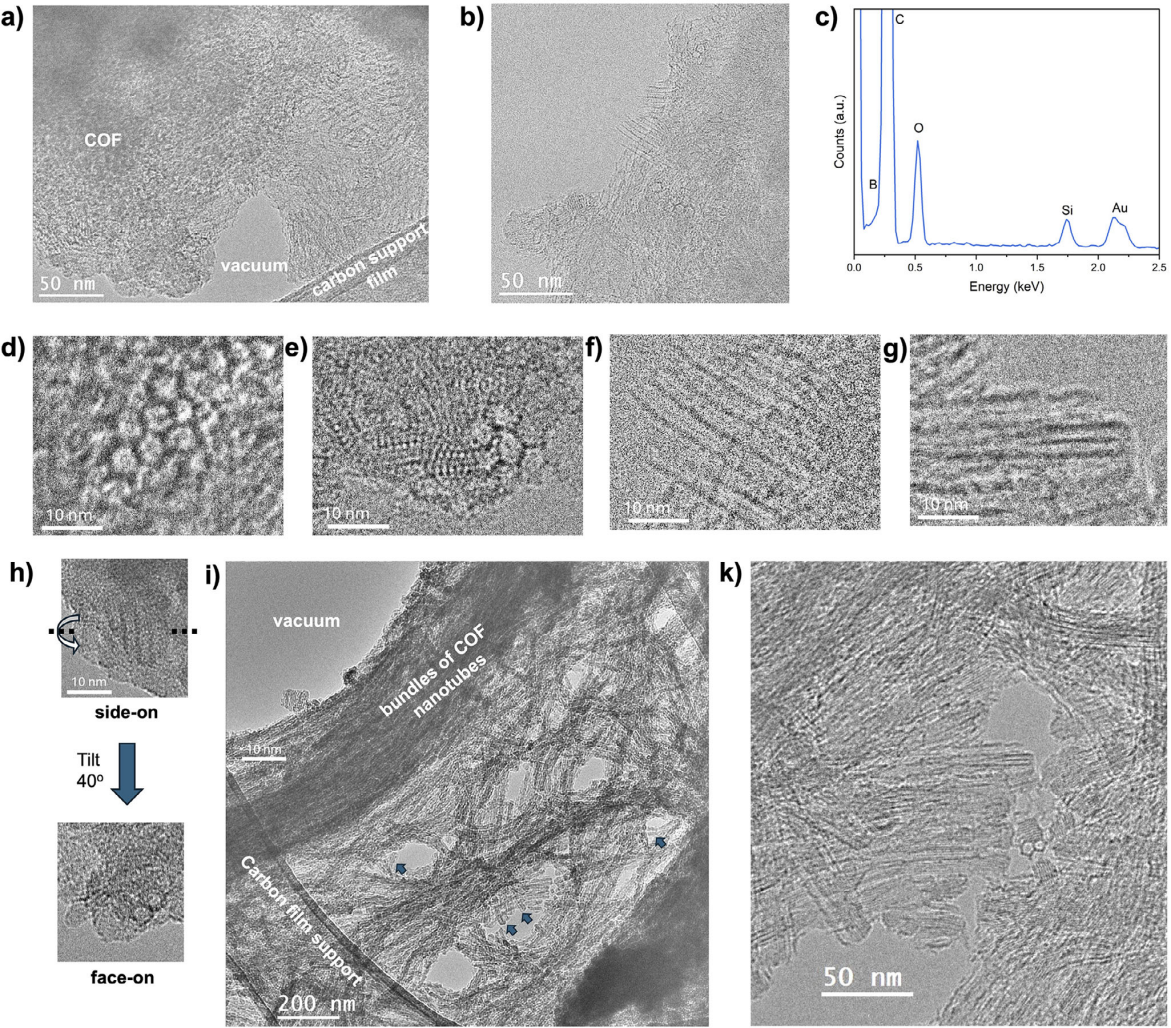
200 kV TEM analysis of Joa‐COF‐1 a) and b) Large field of view HR‐TEM images of COF particles showing areas with different orientations of 6 nm channels (side‐on and face‐on orientations). c) The EDX spectrum of a COF particle shows C and O peaks (the B peak overlaps with the C peak and appears as a shoulder; the Si and Au peaks are from the TEM grid). d), e) face‐on and f), g) side‐on images of hexagonal channels in the COF structure. h) Tilting helps to reveal the relationship between the side‐on and face‐on projections of the same group of channels. i), k) Bundles of COF nanotubes are formed by disassembling Joa‐COF‐1 particles by ultrasound (arrows in i) indicate sections of single COF nanotubes protruding from the bundles).
